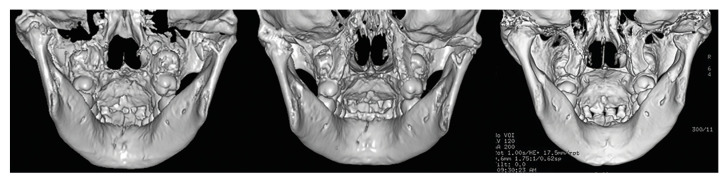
CT images of a case with comminution of condylar fractures that underwent surgery to remove fracture fragments (left: before surgery; middle: 1 week after surgery; right: 6 months after surgery).
